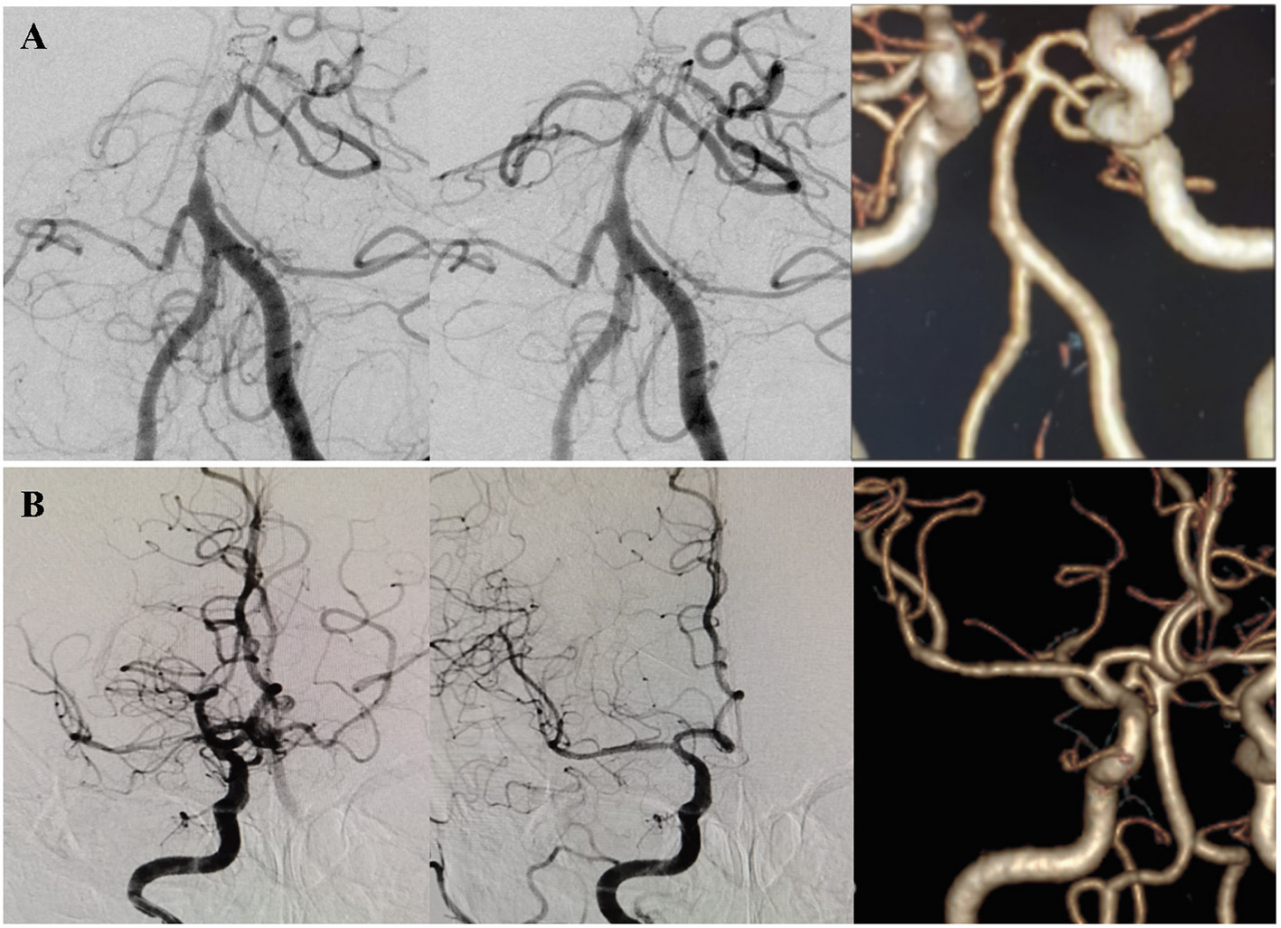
Two patients with no significant ISR by CTA. (A) A patient presented with dizziness and diplopia for 2 months. DSA showed a high-grade (>90%) stenosis at the middle segment of the basilar artery, classified as Mori B type. The lesion was dilated with a 2 × 9 mm Gateway balloon and implanted a 4.5 × 14 mm Enterprise stent. Postoperative DSA showed the degree of stenosis decreased to 30%. CTA after 54.5 months of the procedure showed no significant ISR. (B) A patient presented with left limb weakness and dysarthria for 10 days due to a high-grade (>90%) stenosis at the M1 segment of the right MCA. The Mori classification was type C. The lesion was dilated with a 2 × 9 mm Gateway balloon and implanted a 4.5 × 22 mm Enterprise stent. Postoperative DSA showed the degree of stenosis decreased to 5%. CTA after 23 months of the procedure showed no significant ISR.
